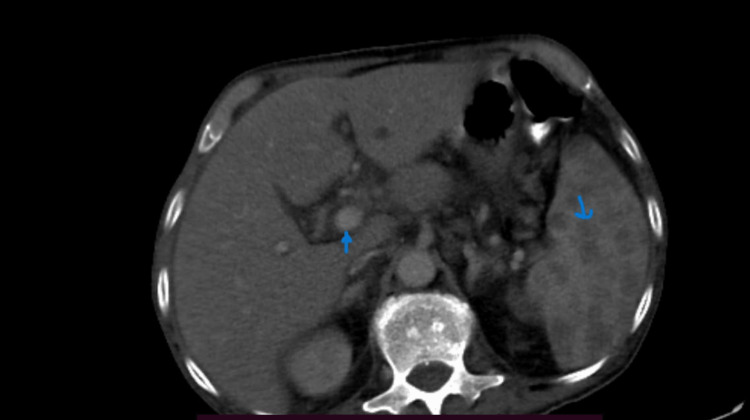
CT image showing multiple multiple liver abscesses and splenic abscesses
